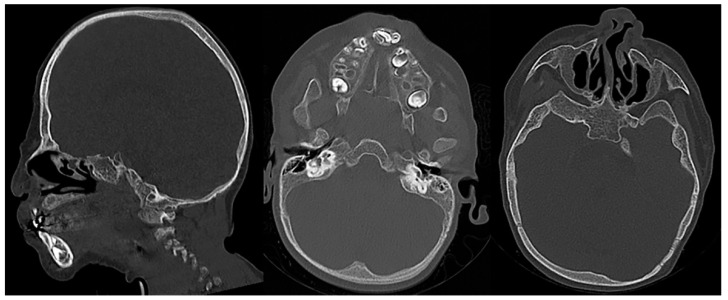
Sagittal and axial CT images (bone window) of a child with confirmed Langer-Giedion syndrome show the widening of the diploic space of the bony calvarium, extensive maxillofacial deformity with maxillary and mandibular hypoplasia, and a bulbous deformed nasal tip.
